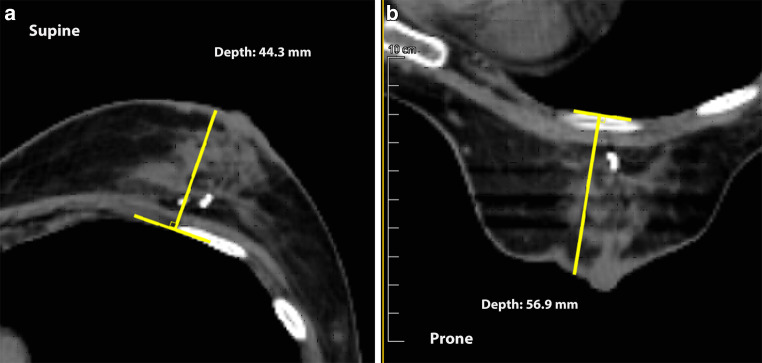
Measurement of breast depth supine (a) and prone (b). The depth was measured on the CT slice through the nipple using an on-screen adjustable T‑square ruler, recording the largest distance from the breast contour to the base set tangent to the pleura. If the breast point falls on the nipple, the measurement was performed flush with the areola
